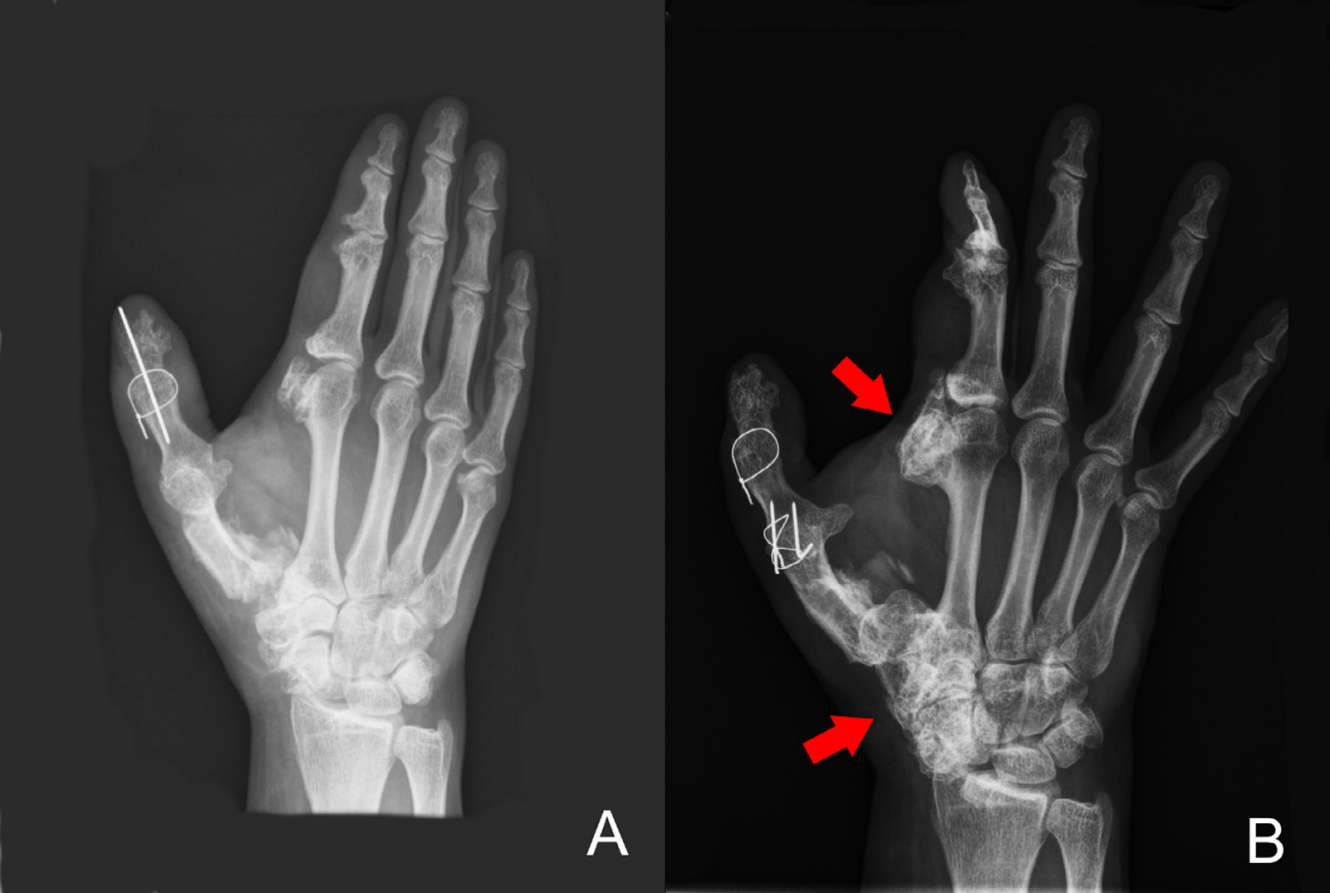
Conventional radiograph of the right hand of case 4. Status after soft tissue debulking surgery of the right thumb in 1989, additional soft tissue debulking, shortening of the proximal phalanx, and arthrodesis of the IP joint and MCP joint of the thumb in 2009, and correction osteotomy of the thumb in 2009. The current radiographs are showing progression of juxta-articular new bone formation between age 38 (8A) and age 47 (8B). In particular, the thumb and index finger (upper arrow) and the scaphoid, trapezium, and trapezoid (bottom arrow) show severe progression.
